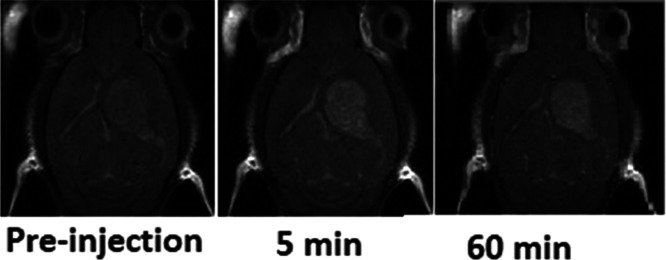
Top: Axial *T*_1_w MRI for the follow-up
